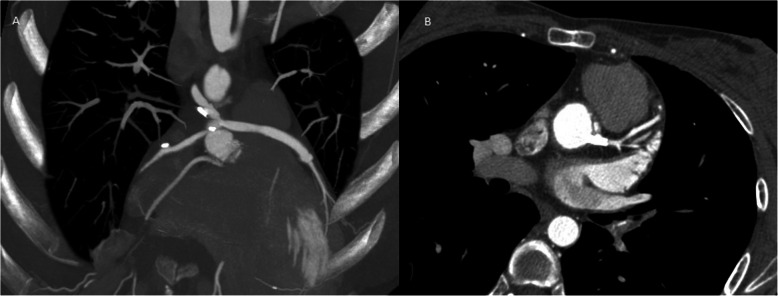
a CT-coronary angiogram, three months after revascularization showing patent stent
